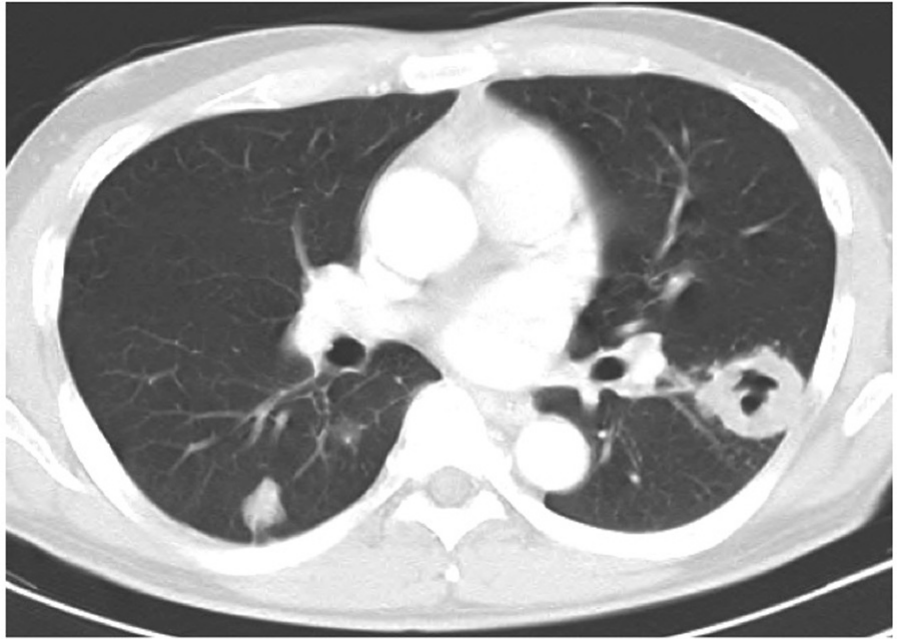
Chest CT in December 2006 showed cavitation of the nodule in the left lung field.
